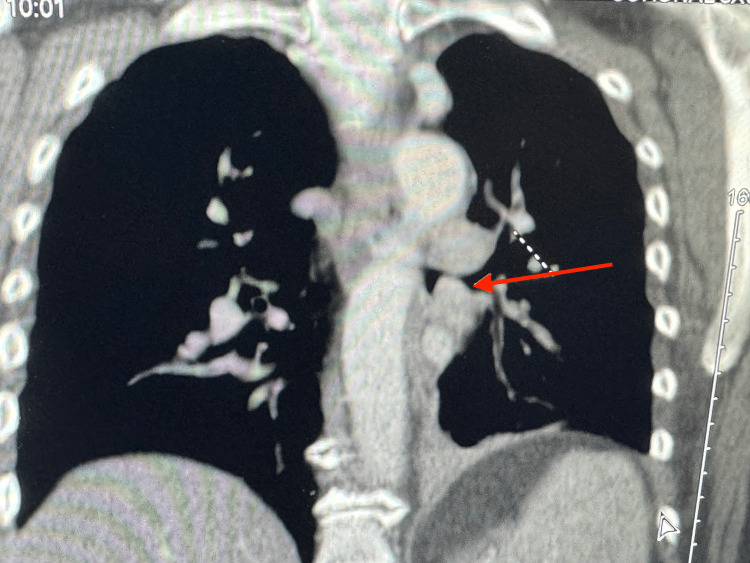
Coronal view CT scan showing a left lower lobe tumor (arrow)
